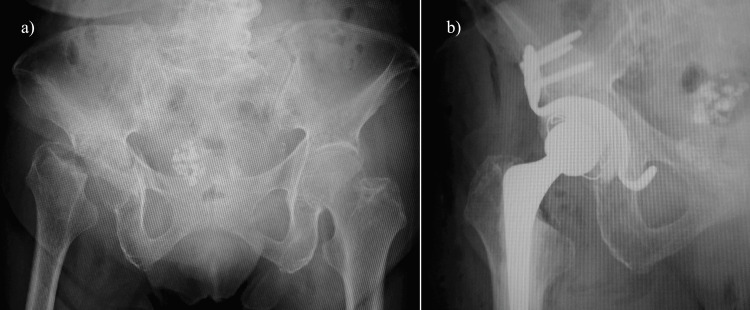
Radiographs of case 2: an 82-year-old female patient a) Preoperative radiograph and b) Postoperative radiograph
